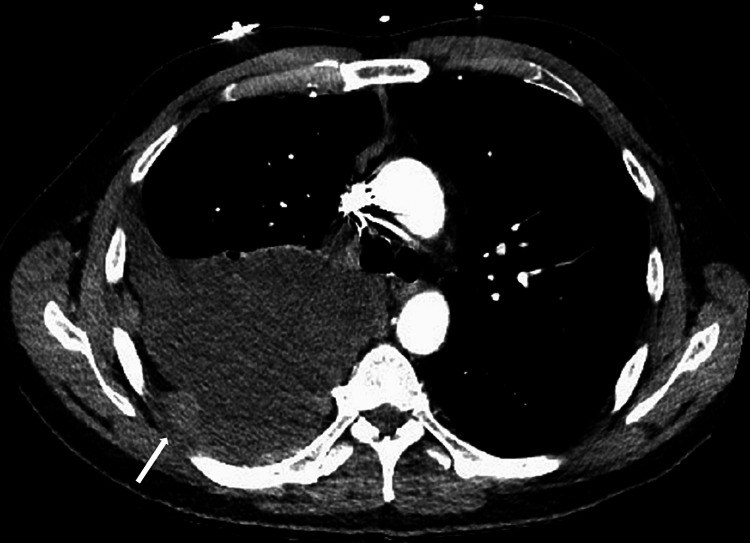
CT chest with contrast demonstrating diffuse enhancing pleural thickening and nodularity on the right; a 2.8 x 2.9cm nodular thickening noted laterally
